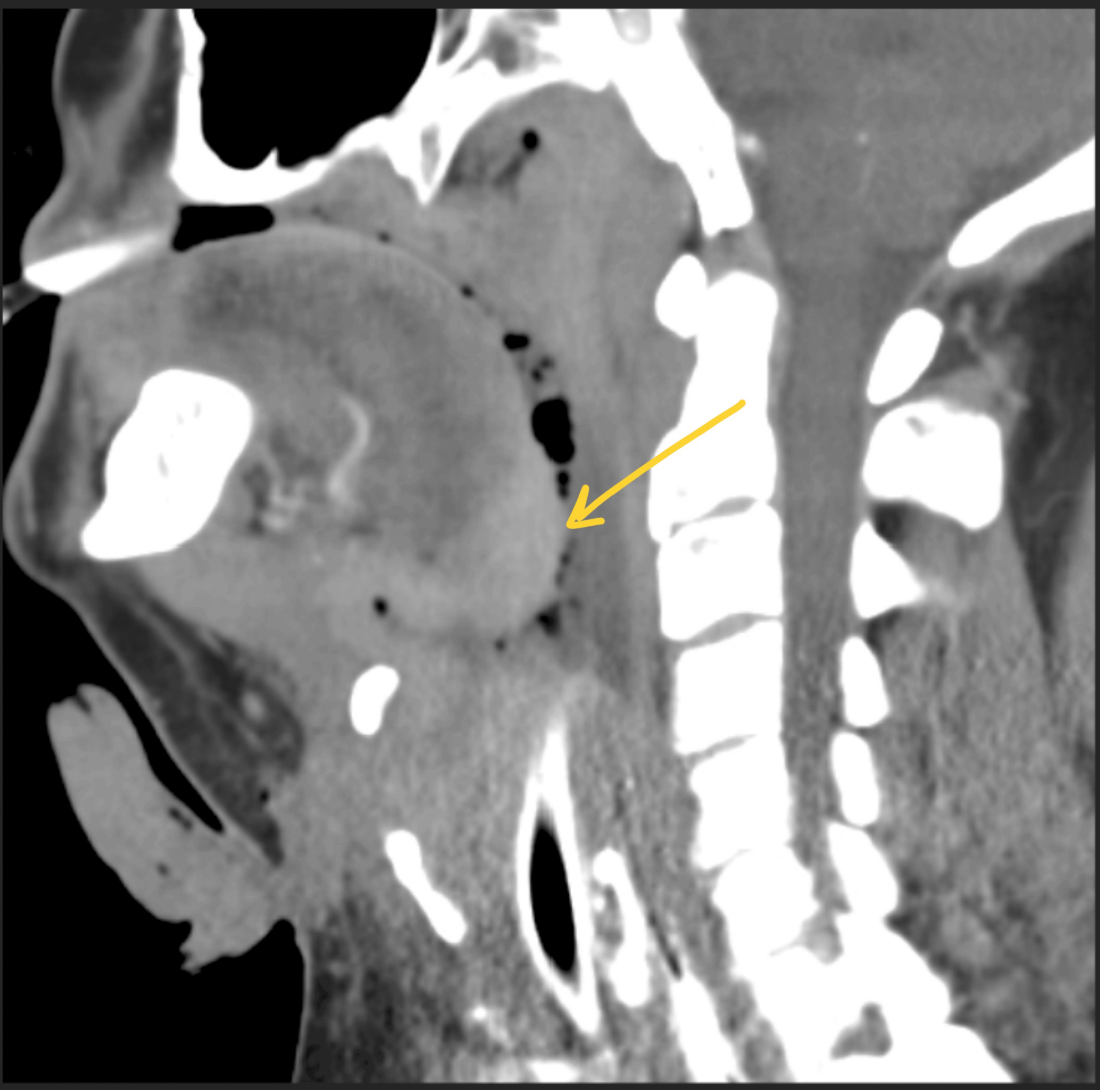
Sagittal view of CT soft tissue neck with contrast
